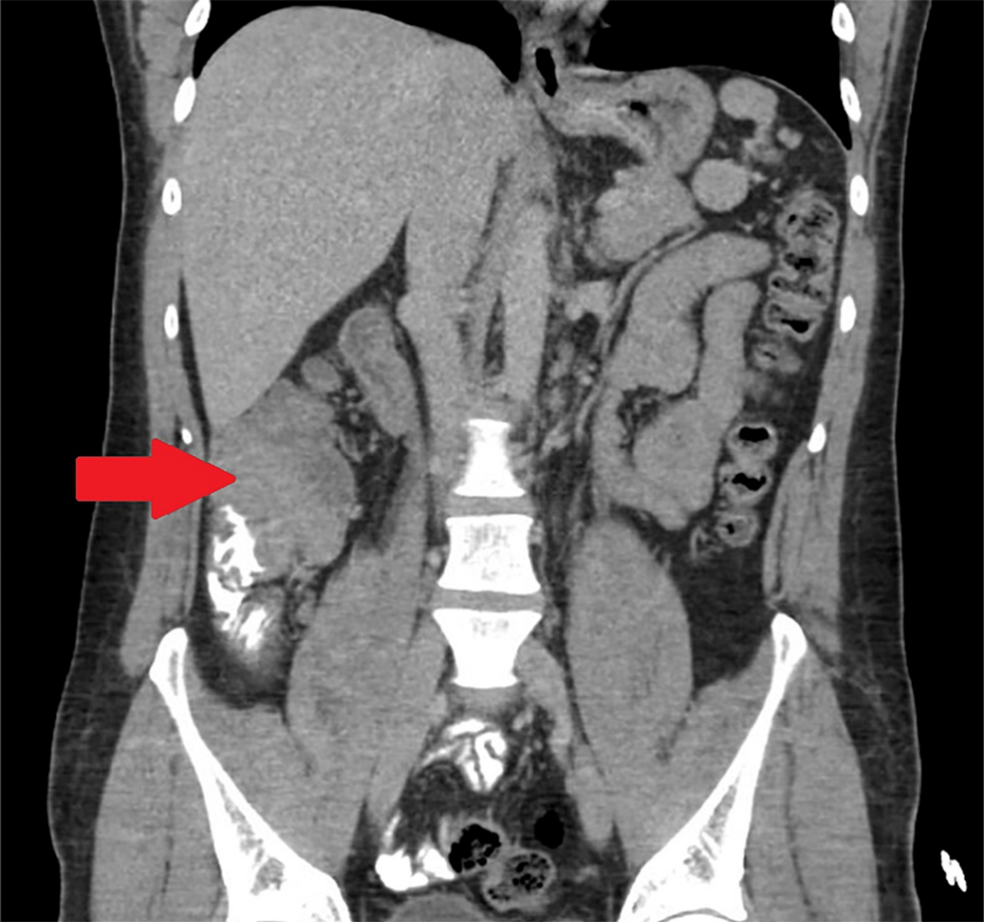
Coronal view of CT scan of the abdomen and pelvis. Red arrow points towards the cecal mass.
